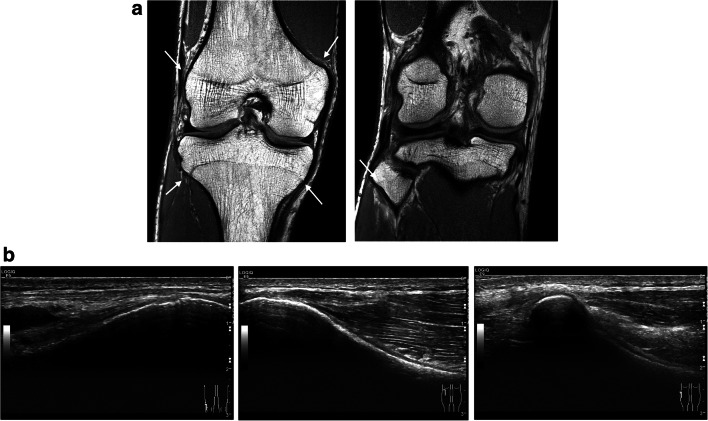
Right knee of a 17-year-old boy showing closed physeal plates (arrows) on a coronal T1-weighted MRI (a) and longitudinally oriented US images of the lateral distal femur (right image), the lateral proximal tibia (middle image), and the proximal fibula (left image). On the US, note the closed echogenic line of the cortical bone (US-S3)
